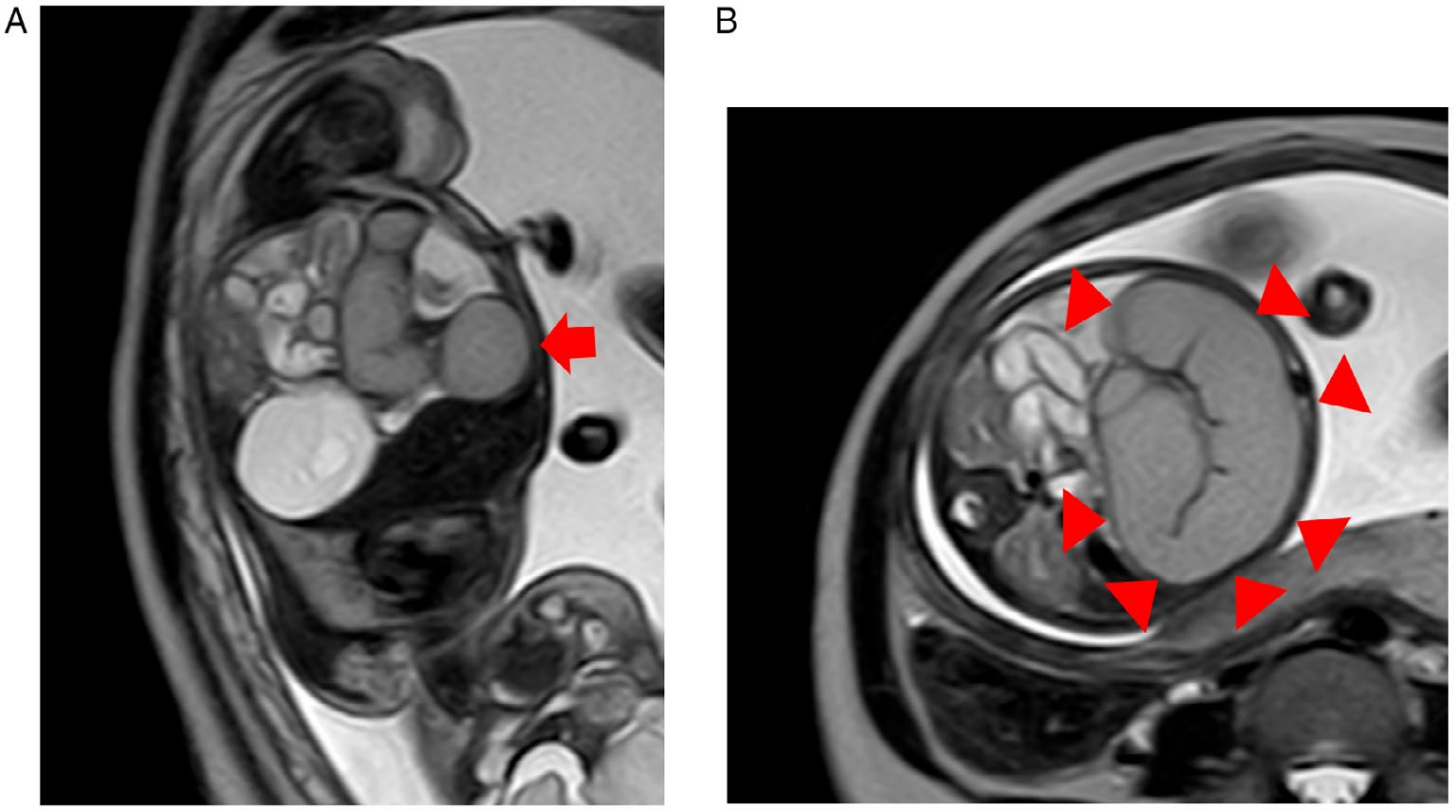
(A) Fetal MRI (T2‐weighted image) shows low signal intensity within the dilated small intestine (arrow), in contrast to adjacent normal bowel (“black‐and‐white sign”). (B) The twisted bowel loop (arrowhead) appears oval‐shaped with wall thickening—resembling a “coffee‐bean sign.”
